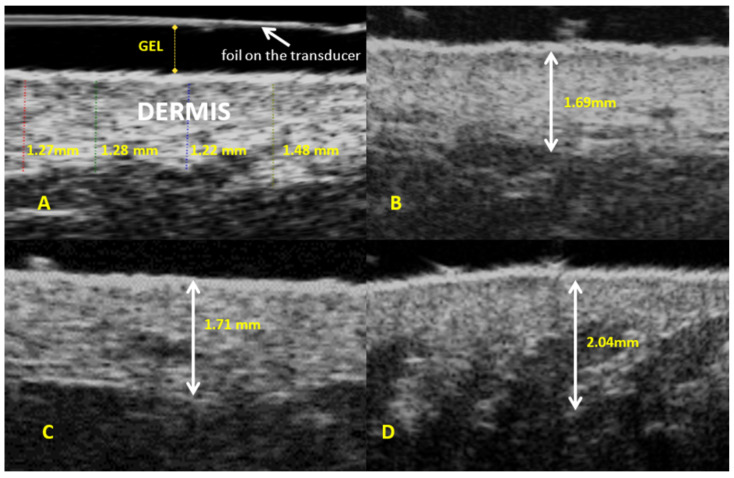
Ultrasound assessment of dermis (A). Dermis thickness: (B)—group 1; (C)—group 2; and (D)—group 3.
